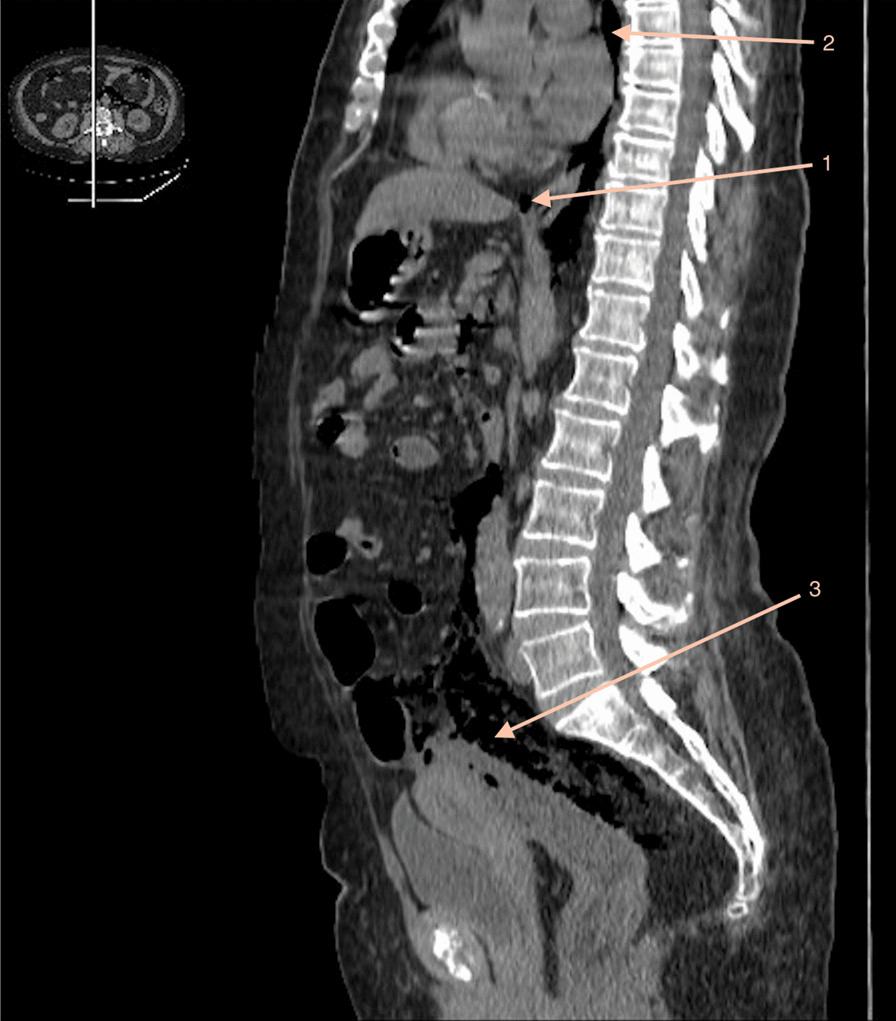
CT scan sagittal view showing pneumoperitoneum [1], pneumoretroperitoneum [3] and pneumomediastinum [2]. Arrow 1 points to pneumoperitoneum, arrow 2 points to pneumomediastinum, arow 3 points to pneumoretroperitoneum
